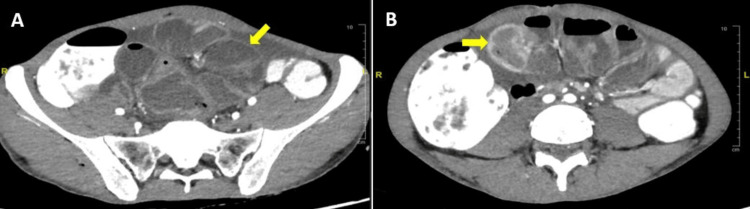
Axial slice of abdominal CT with contrast showing clumped small bowel loops in the center of the abdomen encapsulated by an enhancing membrane (yellow arrow) (A). Axial slice of abdominal CT with contrast showing a congregate of small bowel loops giving the appearance of an accordion or “concertina-like pattern” (yellow arrow) (B).
